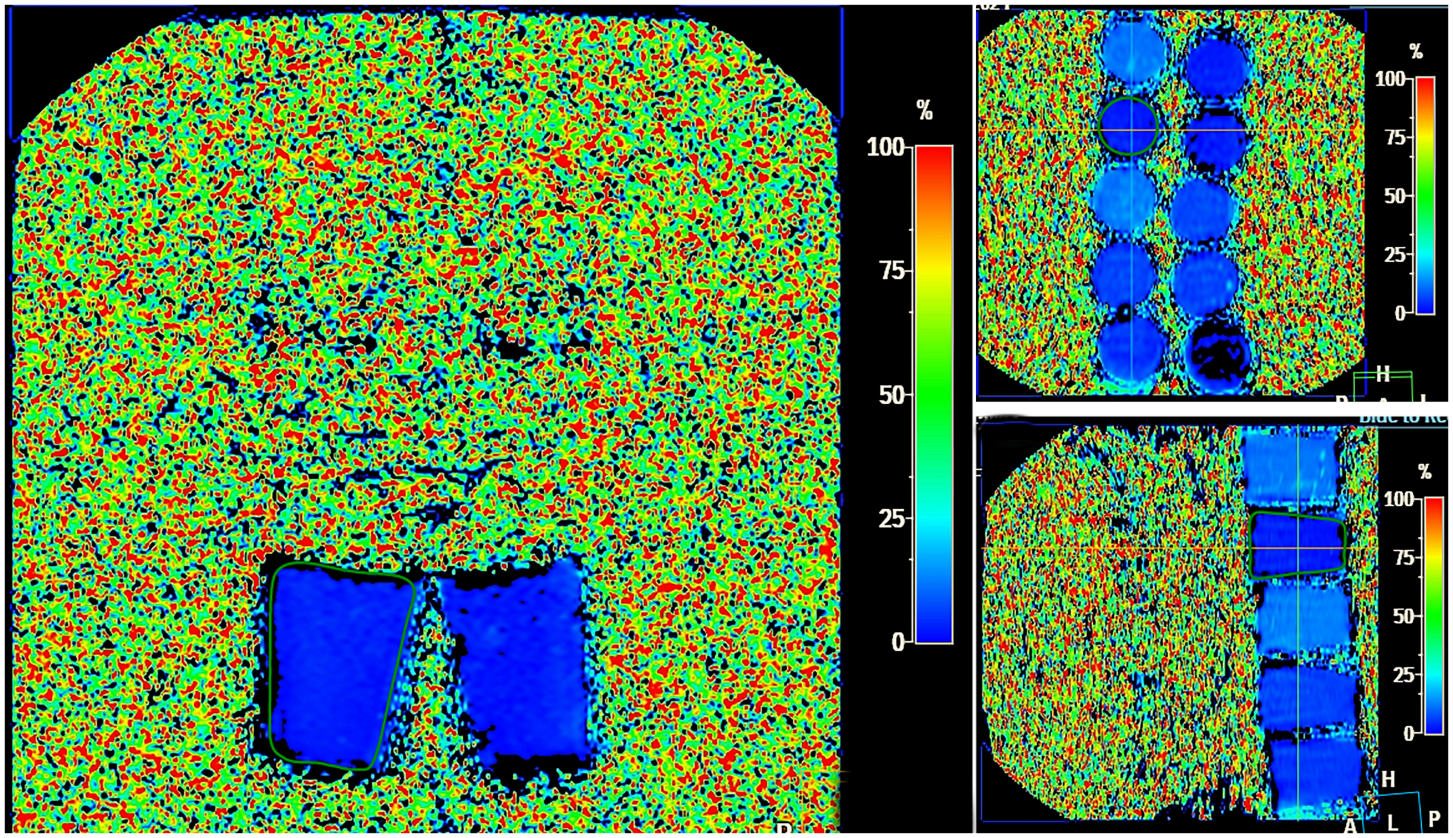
MRI steatometry mDIXON_Quant, Liver Health postprocessing—color map showing different degrees of fat in LFP in the FF range from 0%−100% (shades from dark blue–light blue).
